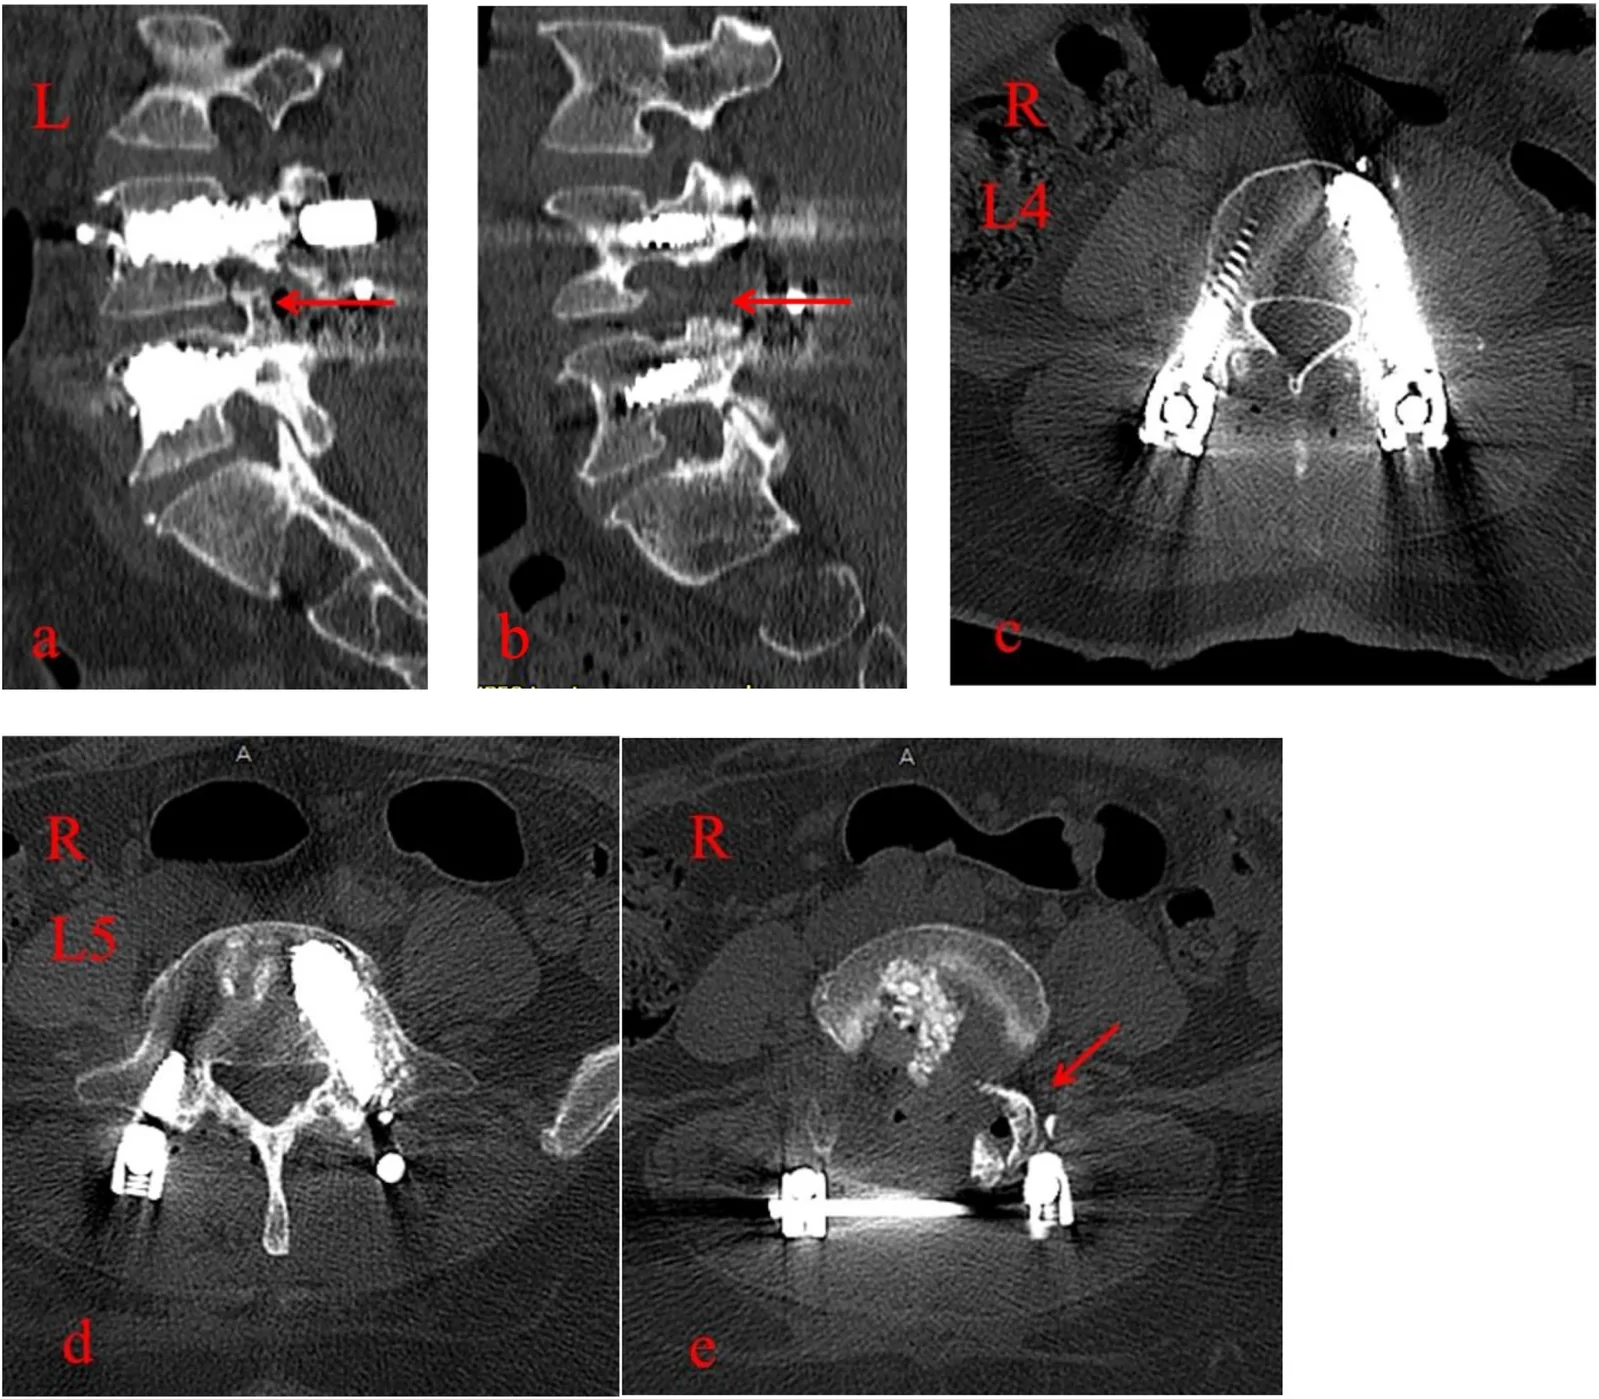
A postoperative CT demonstrated satisfactory segmental reduction and optimal positioning of the interbody cage and pedicle screws with no obvious cement leakage (a–e). Sagittal and axial images showed residual bony tissue of the left SAP resulting in secondary foraminal stenosis (a,e), in contrast to the surgically enlarged neuroforamen on the right (b).
